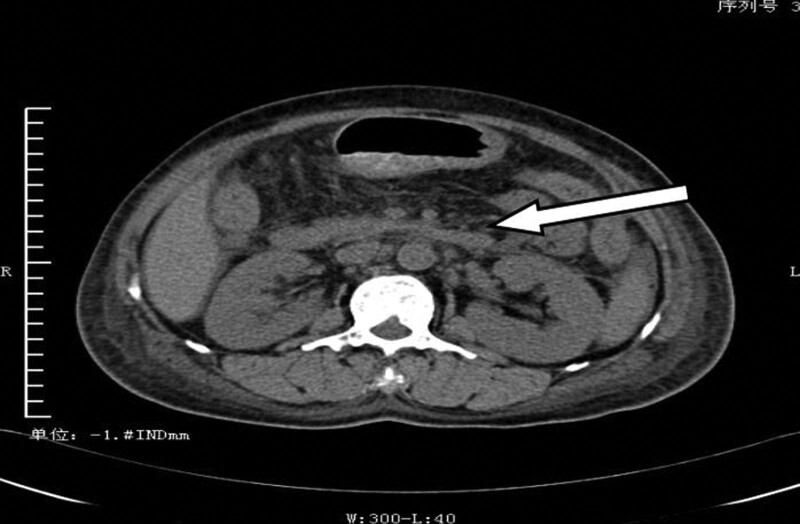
The abdominal CT scan reveals enlargement of the body skin, abdominal cavity, and pelvic lymph nodes. CT = computed tomography.
